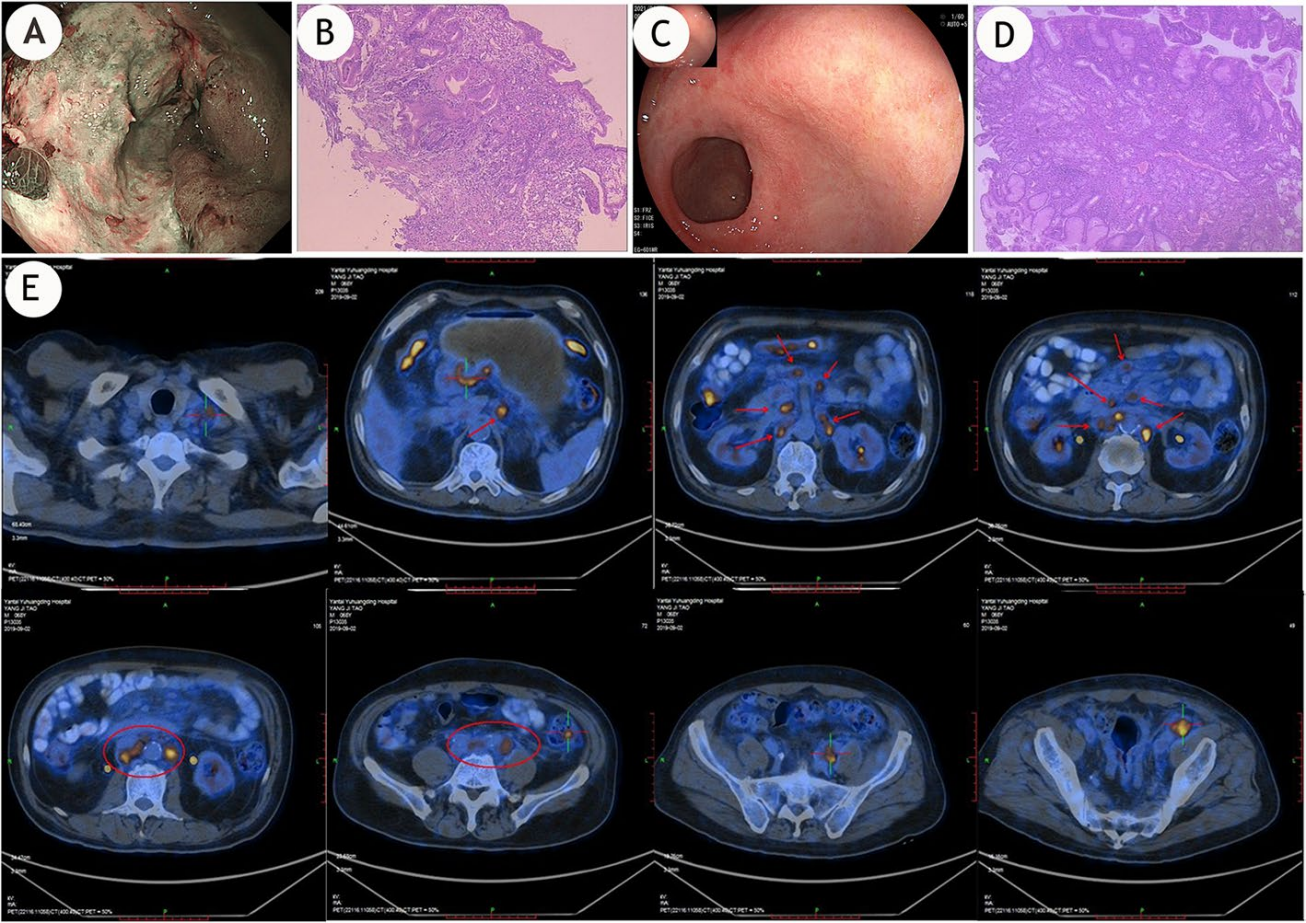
Baseline data of the patient. A Gastric ulcer found during gastroscopy. B Pathological findings (gastric antrum) adenocarcinoma, part of which showed signet ring cell carcinoma differentiation. C After more than a year of treatment, the gastric ulcer lesions (cancer) disappeared in April 2021. D The antrum mucosal tissue is inflammatory, and the glandular epithelium presents mild intestinal metaplasia. E PET/CT showed gastric cancer with multiple lymph node metastases in the left supraclavicular fossa, lesser omentum sac, retroperitoneal, mesenteric root, and left iliac artery
